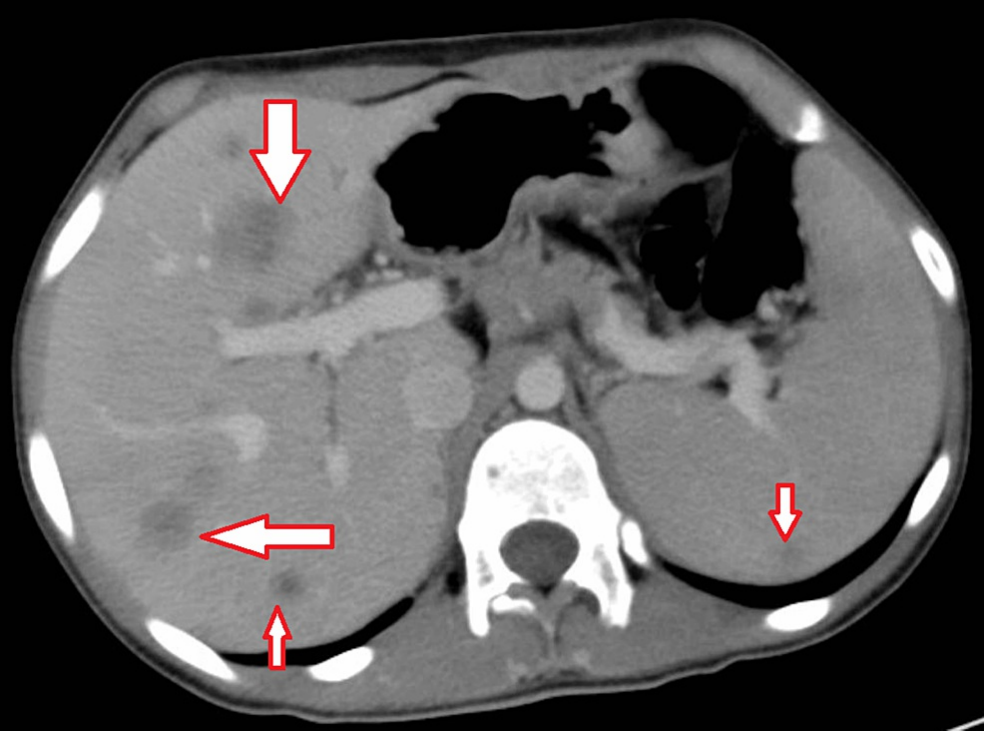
CT abdominal scan showing the presence of multiple liver lesions and a possible splenic lesion, which could reflect metastasis
